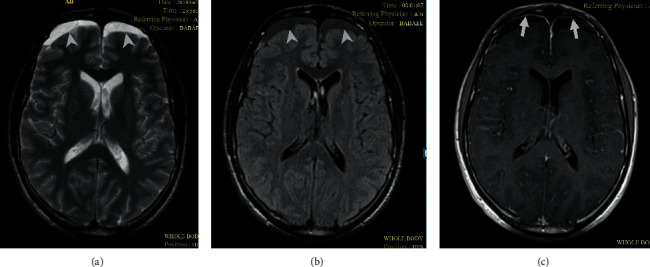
Brain magnetic resonance imaging indicative of bilateral subdural effusion (arrowheads in (a, b)) and diffuse leptomeningeal enhancement predominantly on the anterior surface of both hemispheres (arrows in (c)).
